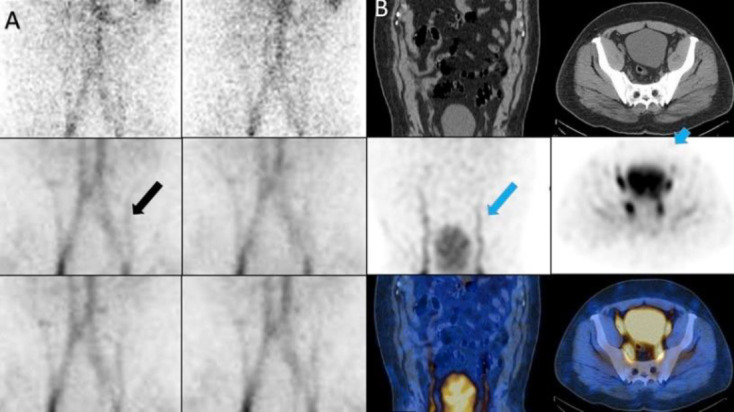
Inferior epigastric veins on planar (A) and SPECT/CT (B)
